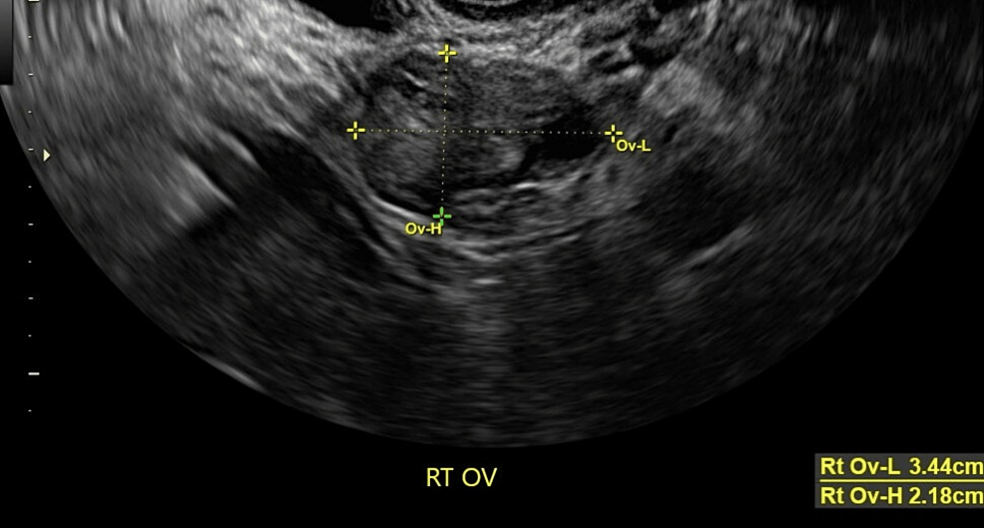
Ultrasound image showing the right ovary measuring 3.4 x 2.1 cm with a regular appearance
